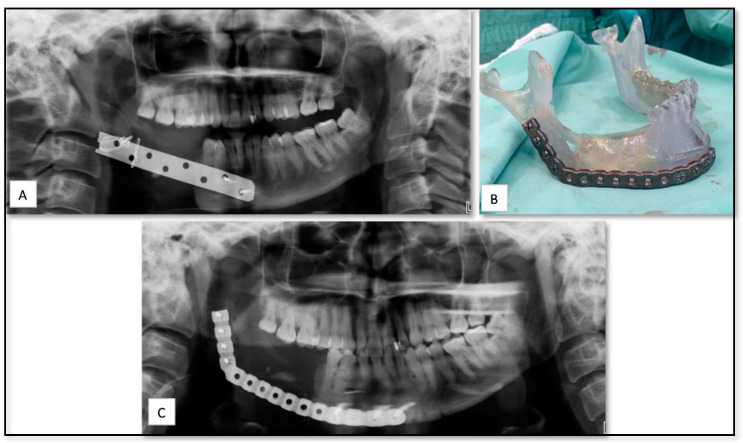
(A) Panoramic radiograph showing right mandibular defect with the old bone plate. (B) Stereolithographic (SLA) model and the prebent reconstruction plate. (C) Post-operative panoramic radiograph showing the installed reconstruction plate.
